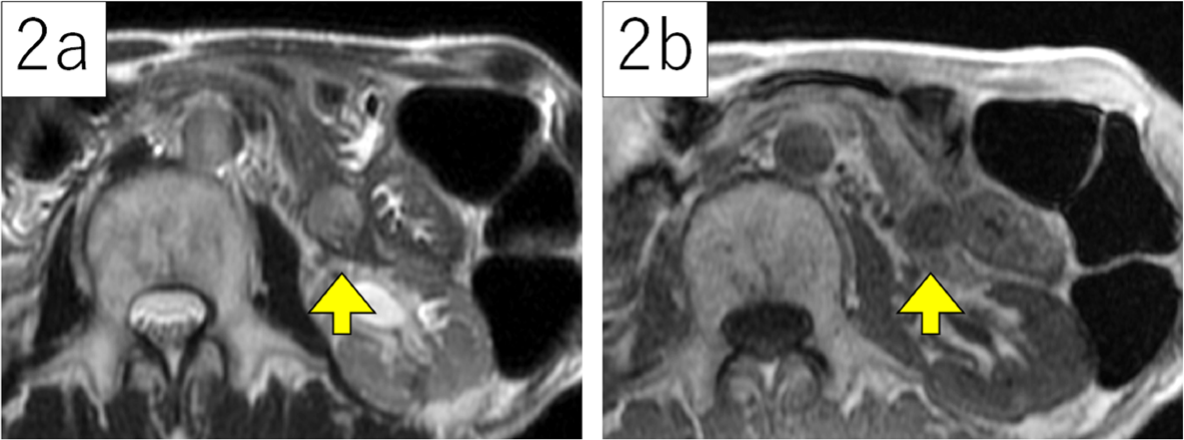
Magnetic resonance imaging (MRI) showed high signal intensity in T2WI (2a) and low intensity in T1WI (2b), each indicated with arrowheads
